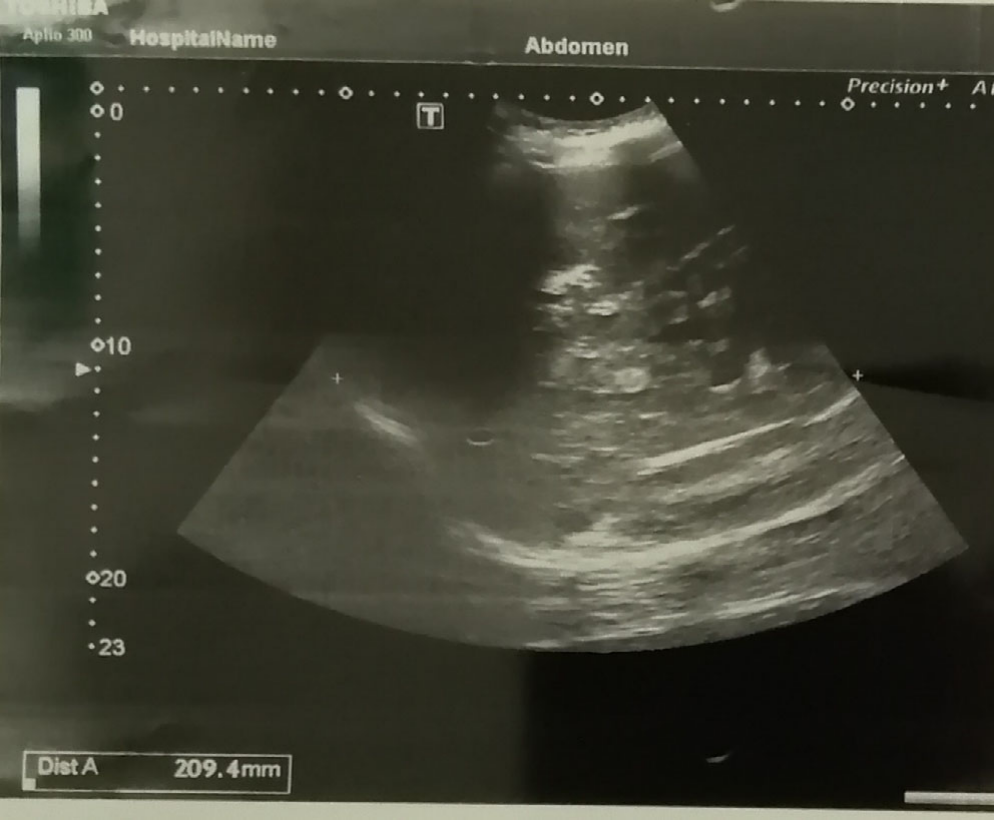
Abdominal ultrasonography.
